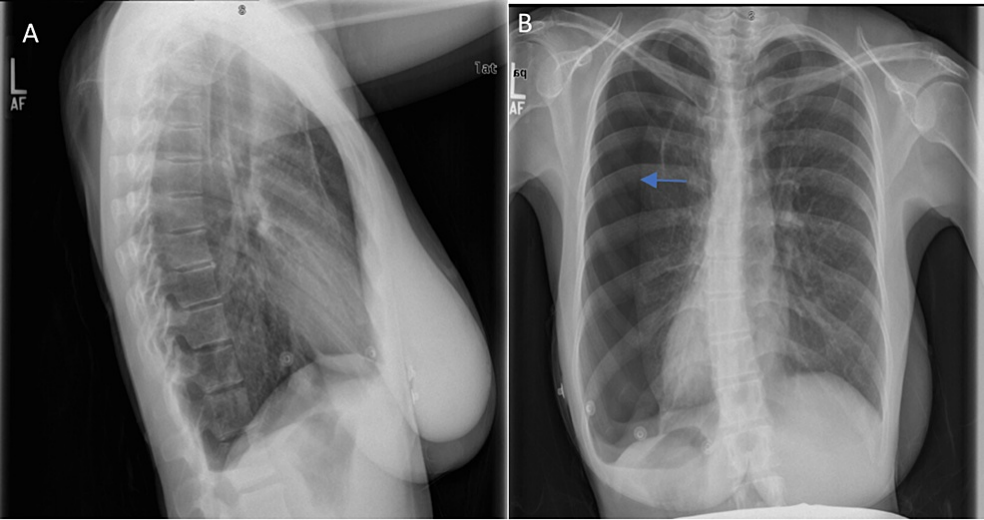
Initial Chest X-ray from the Emergency Room Emergency Room Chest X-ray positive for large left-sided hydropneumothorax (arrow), with mild mediastinal shift to the right. Figure 1A shows a lateral X-ray and Figure 1B is a posterior-anterior X-ray. A large left-sided hydropneumothorax is highlighted by the arrow.
